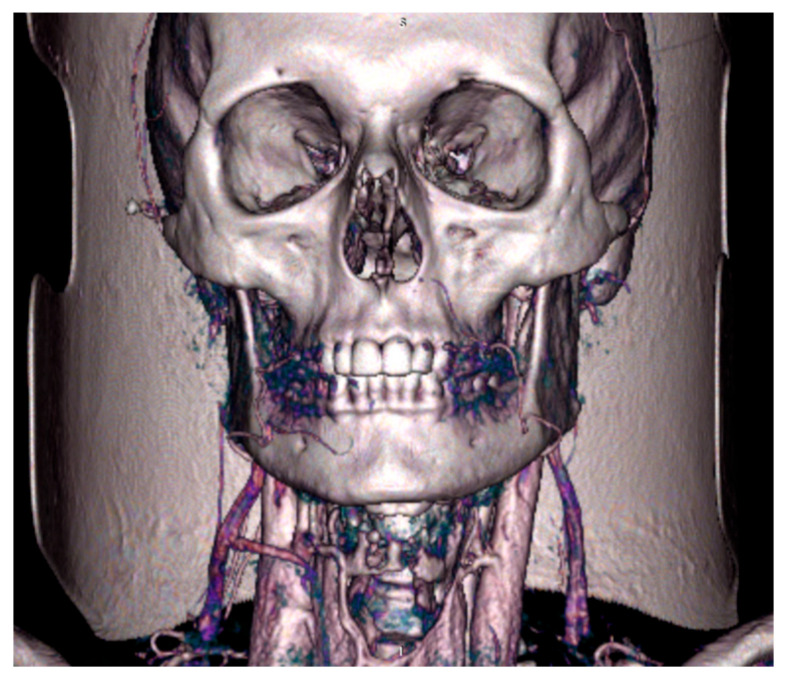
Three-dimensional reconstruction of multiphasic computed tomography angiography of the head and neck. The reconstruction was performed using a Canon Aquilion ONE dynamic 640-slice CT scanner. The image shows the bony structures, major arteries (external carotid artery and its branches), and the pterygoid venous plexus (PVP) before segmentation.
